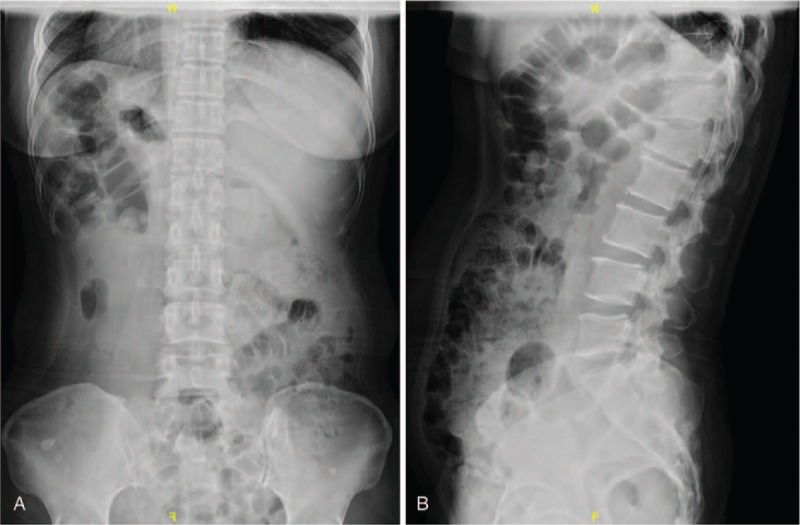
(A and B) Preoperative x-rays revealing vertebral fracture of L1 with high suspicion of metastatic spinal tumors.
